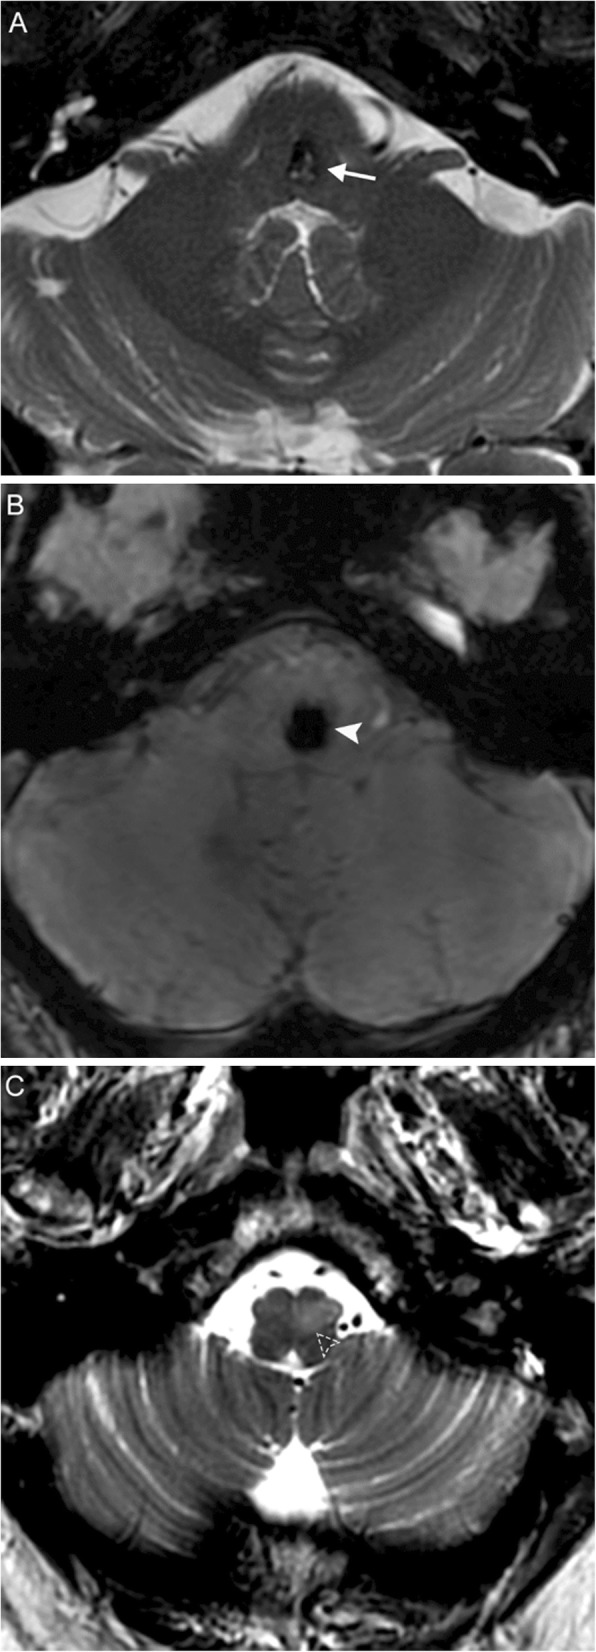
Hypertrophic olivary degeneration. A 69-year-old man with history of hypertension presenting with new onset palatal myoclonus, hoarse voice, and ataxia. Axial T2 (a) and susceptibility-weighted (b) MR images reveal a lesion at the left parasagittal pontomedullary junction at the level of the facial colliculus demonstrating bubbly high T2 signal internally surrounded by a rim of low T2 signal intensity (arrow) and associated blooming (solid arrowhead), compatible with a cavernous malformation. Thin section axial T2 image (c) reveals hyperintensity and expansion of the left inferior olivary nucleus (dashed arrowhead), consistent with hypertrophic olivary degeneration. Lesions involving the dento-rubro-olivary tract (such as this cavernous malformation) can lead to hypertrophic olivary degeneration
